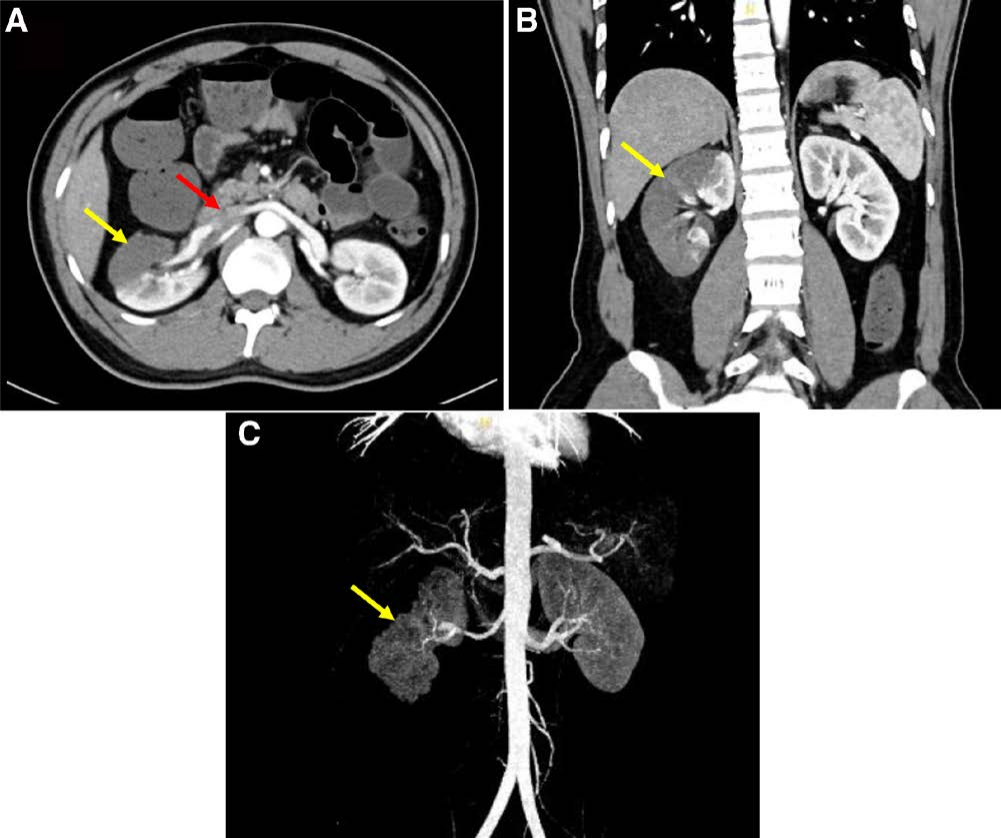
Contrast-enhanced CT image of the patient showing a right renal infarction. (A) Axial section; (B) sagittal section; (C) vascular reconstruction. A patchy low-density area in the right kidney (yellow arrow). A strip-like low-density area was noted in the right renal artery region (red arrow).
